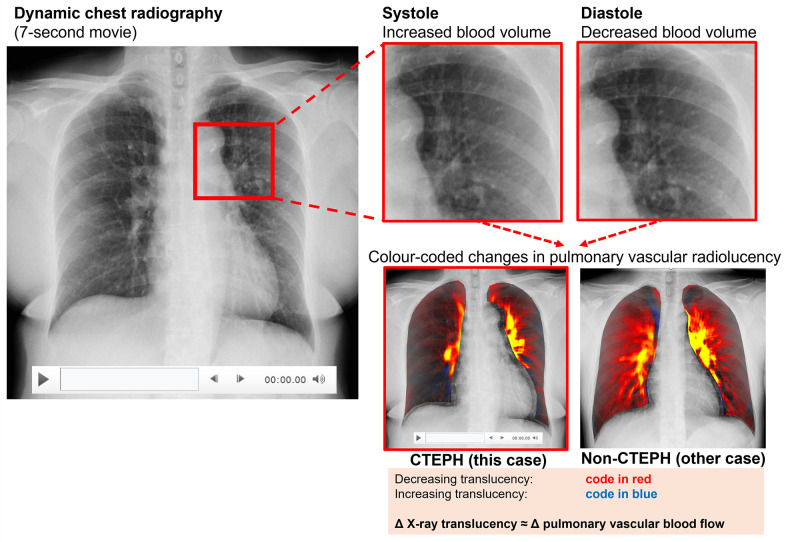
Mechanism of pulmonary circulation analysis based on dynamic chest radiography. Dynamic chest radiography captures cyclic changes in pulmonary blood volume using pulsed X-ray sequences. The dedicated pulmonary circulation analysis program overlays perfusion-surrogate color maps to visualize regional pulmonary blood flow without contrast media. A sample of the DCR-based pulmonary circulation movie is provided in S1 File.
